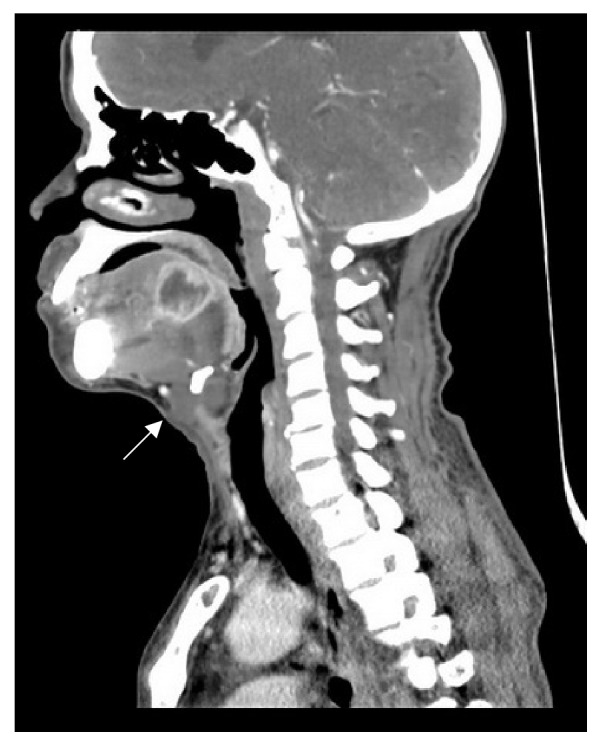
Contrast-enhanced CT scan demonstrated an abscess at left posterior tongue with thyroglossal duct cyst (arrow) was also identified without feature of rim enhancement.
